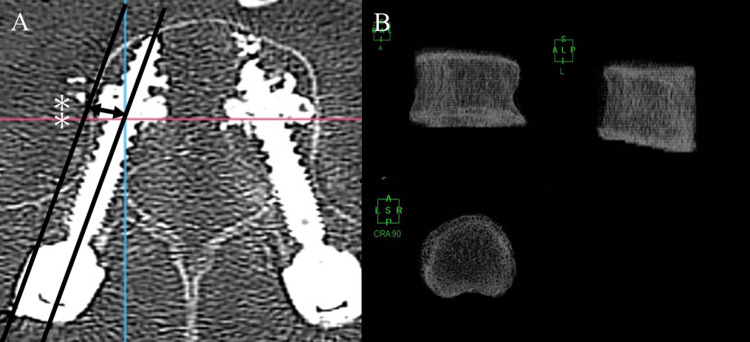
Measurement of imaging parameters (A) The asterisk represents the distance from the tangent line between the vertebral body wall and the screw insertion axis. (B) 3D vertebral body model from preoperative CT images. 3D: three-dimensional, CT: computed tomography
